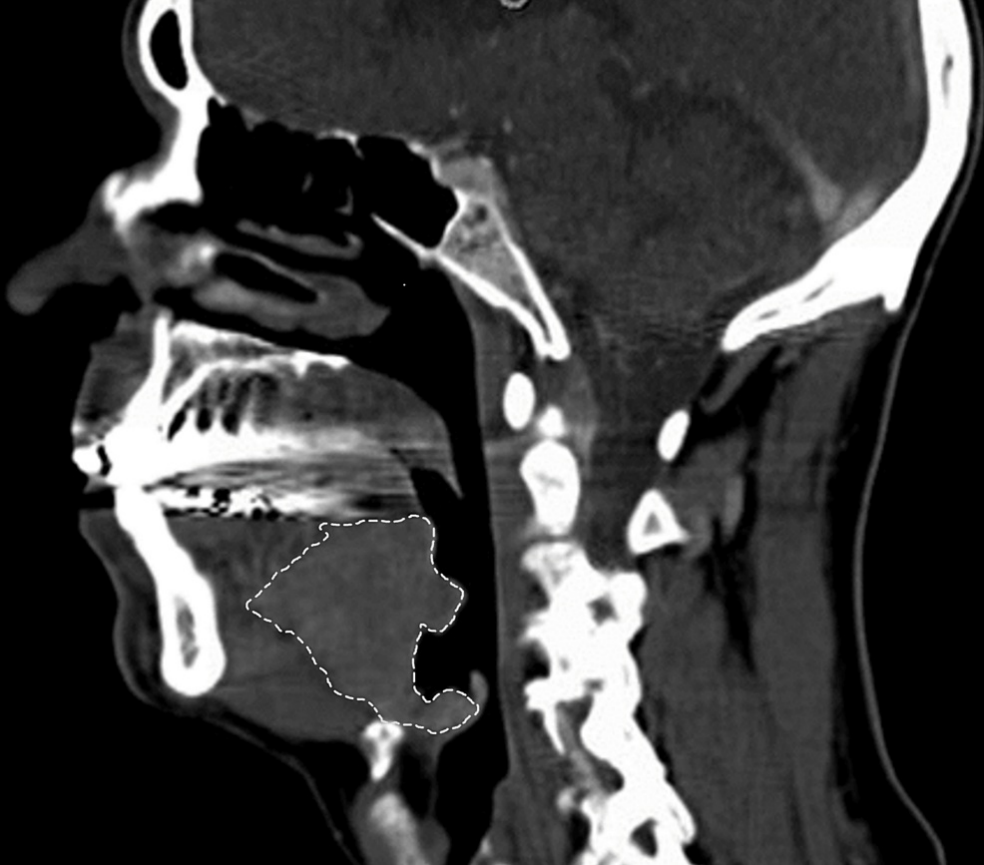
Computed tomography of the neck with contrast revealed a large infiltrative lingual mass suspicious for carcinoma measuring approximately 4.1 x 3.4 x 3.6 cm (dotted line). The mass can be seen involving the lingual surface of the epiglottis and inseparable from portions of the hyoid bone.
